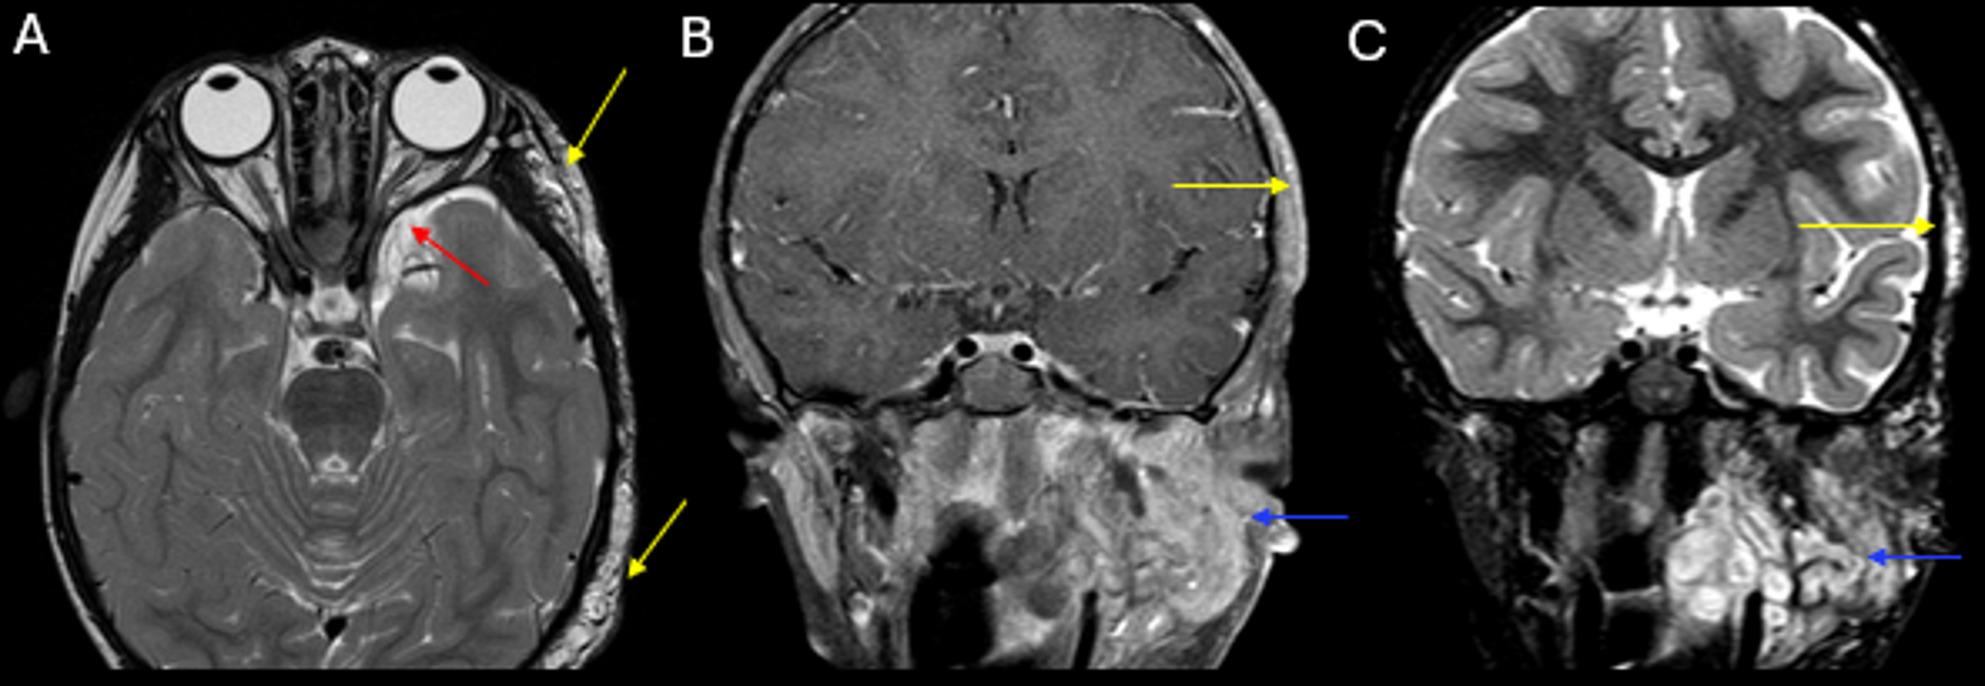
(A) Axial T2-weighted image of the orbits demonstrates dysplasia of the left greater wing of the sphenoid (red arrow). Scalp plexiform neurofibromas with hyperintense T2 signal are noted in the left temporal fossa and posterior occipito-temporal convexity (yellow arrows). (B) Axial T1 fat-suppressed image and (C) coronal STIR image of the sella region show extensive multispatial plexiform neurofibromas centered in the left infratemporal fossa with extension into the parapharyngeal space, masticator space and periauricular region with enhancement and hyperintense STIR (blue arrows in B and C). There is classic “target sign” within the neurofibroma (blue arrow in C)
